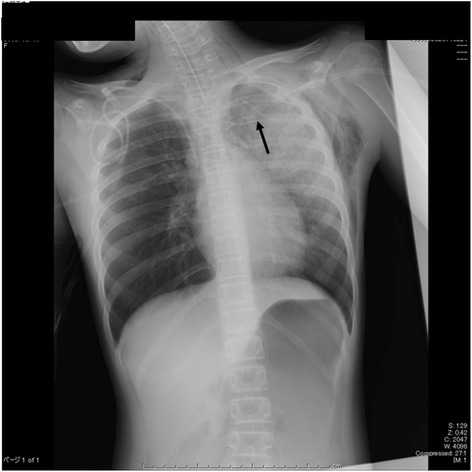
A chest X-ray after emergent chest decompression. The arrow shows the 18G intravenous cannula for chest decompression
